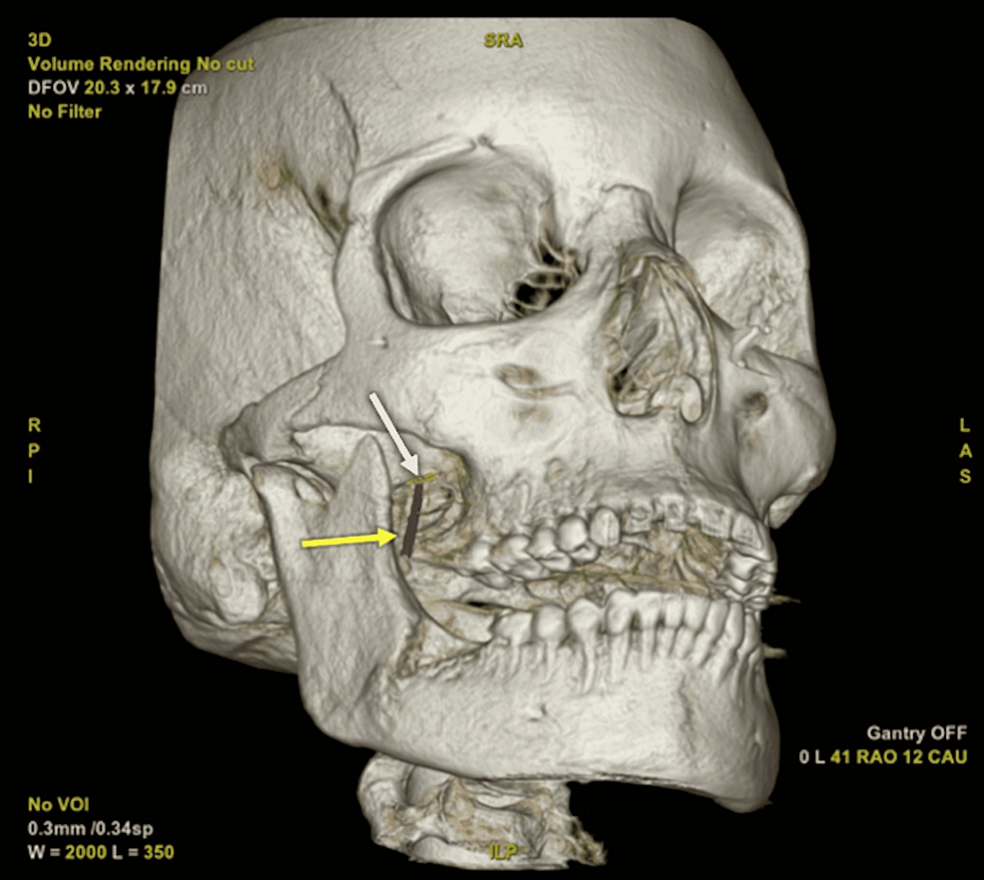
Three-dimensional volume rendered skull from cone beam CT with planned needle trajectory Foramen ovale (white arrow) identified as target for automated generation of needle guidance pathway (yellow arrow).
